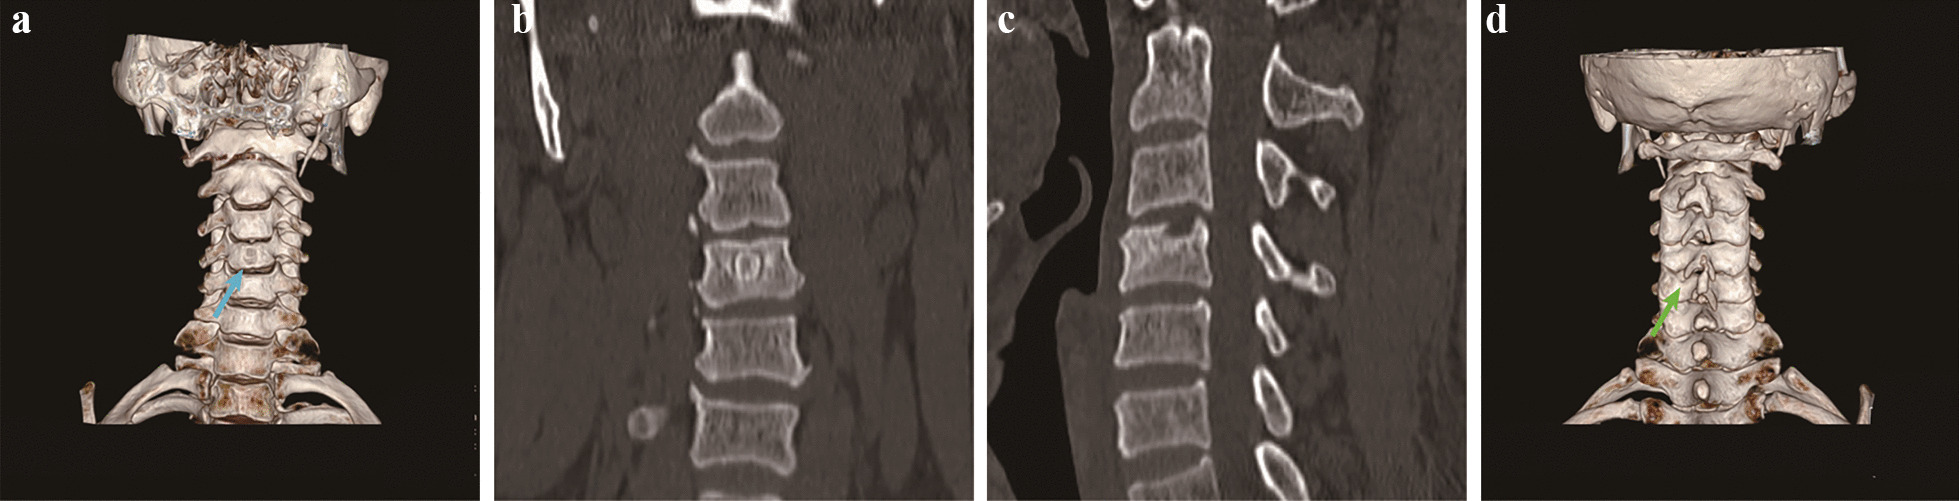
CT images of the patient 6 months after surgery. a CT 3D reconstruction showing the location of the residual entrance to the C4 vertebral channel (blue arrow). b Coronal view showing that the C4 vertebral bone channel is healed and the vertebral body is not collapsed. c Sagittal view showing that the C4 vertebral body bone channel is healed. d CT 3D reconstruction showing that the C5 vertebral plate bone window is healed (green arrow)
